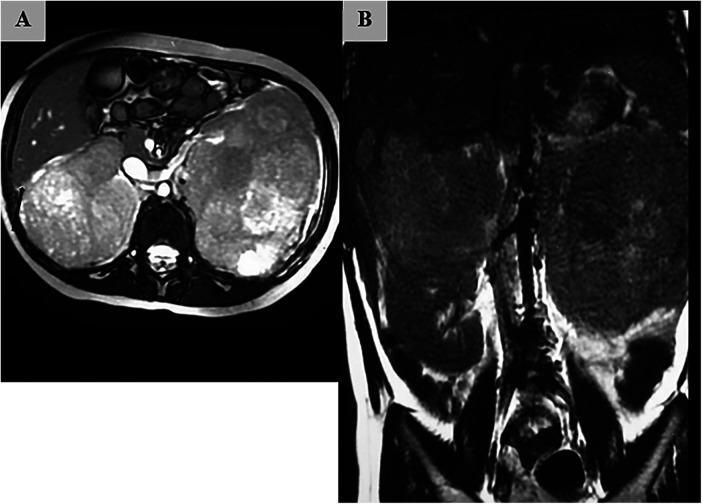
Pre-chemotherapy magnetic resonance imaging (MRI). (A) Axial T2-weighted image demonstrating massive bilateral renal masses, with the right-sided tumor showing effacement of the fat plane with the liver (arrow). (B) Coronal T2-weighted image showing the extensive size of the bilateral tumors, replacing a significant portion of the renal parenchyma.
